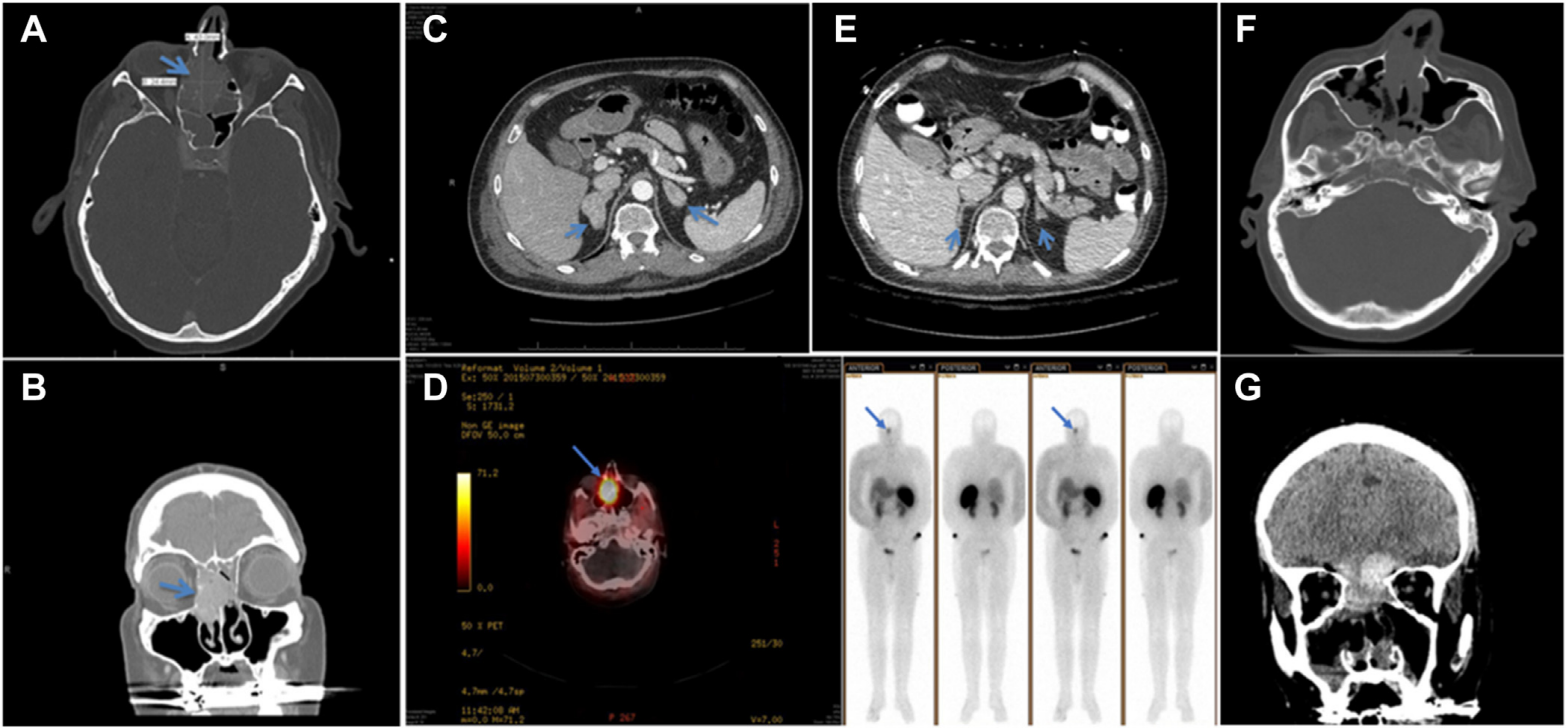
(*A*) Axial and (*B*) coronal noncontrast computed tomography (CT) images of the head demonstrate a heterogeneous soft tissue mass at the anterior skull base extending toward the cribriform plate and into the *right* nasal cavity, involving the ethmoid sinus and eroding the lamina papyracea, resulting in medial displacement of the *right* orbital contents (blue arrows). (*C*) Axial contrast-enhanced CT of the abdomen reveals bilateral adrenal gland enlargement. (*D*) Whole-body single-photon emission computed tomography/computed tomography (SPECT/CT) using indium-111 pentetreotide demonstrates intense radiotracer uptake localized to the biopsy-confirmed esthesioneuroblastoma in the ethmoid sinuses, with no evidence of metastatic octreotide-avid lesions. (*G*) Coronal contrast-enhanced CT scan of the abdomen, performed after surgery, shows normalization in the size of both adrenal glands. (*E*) Coronal and (*F*) axial noncontrast CT images of the paranasal sinuses obtained postoperatively demonstrate complete surgical resection of the tumor.
